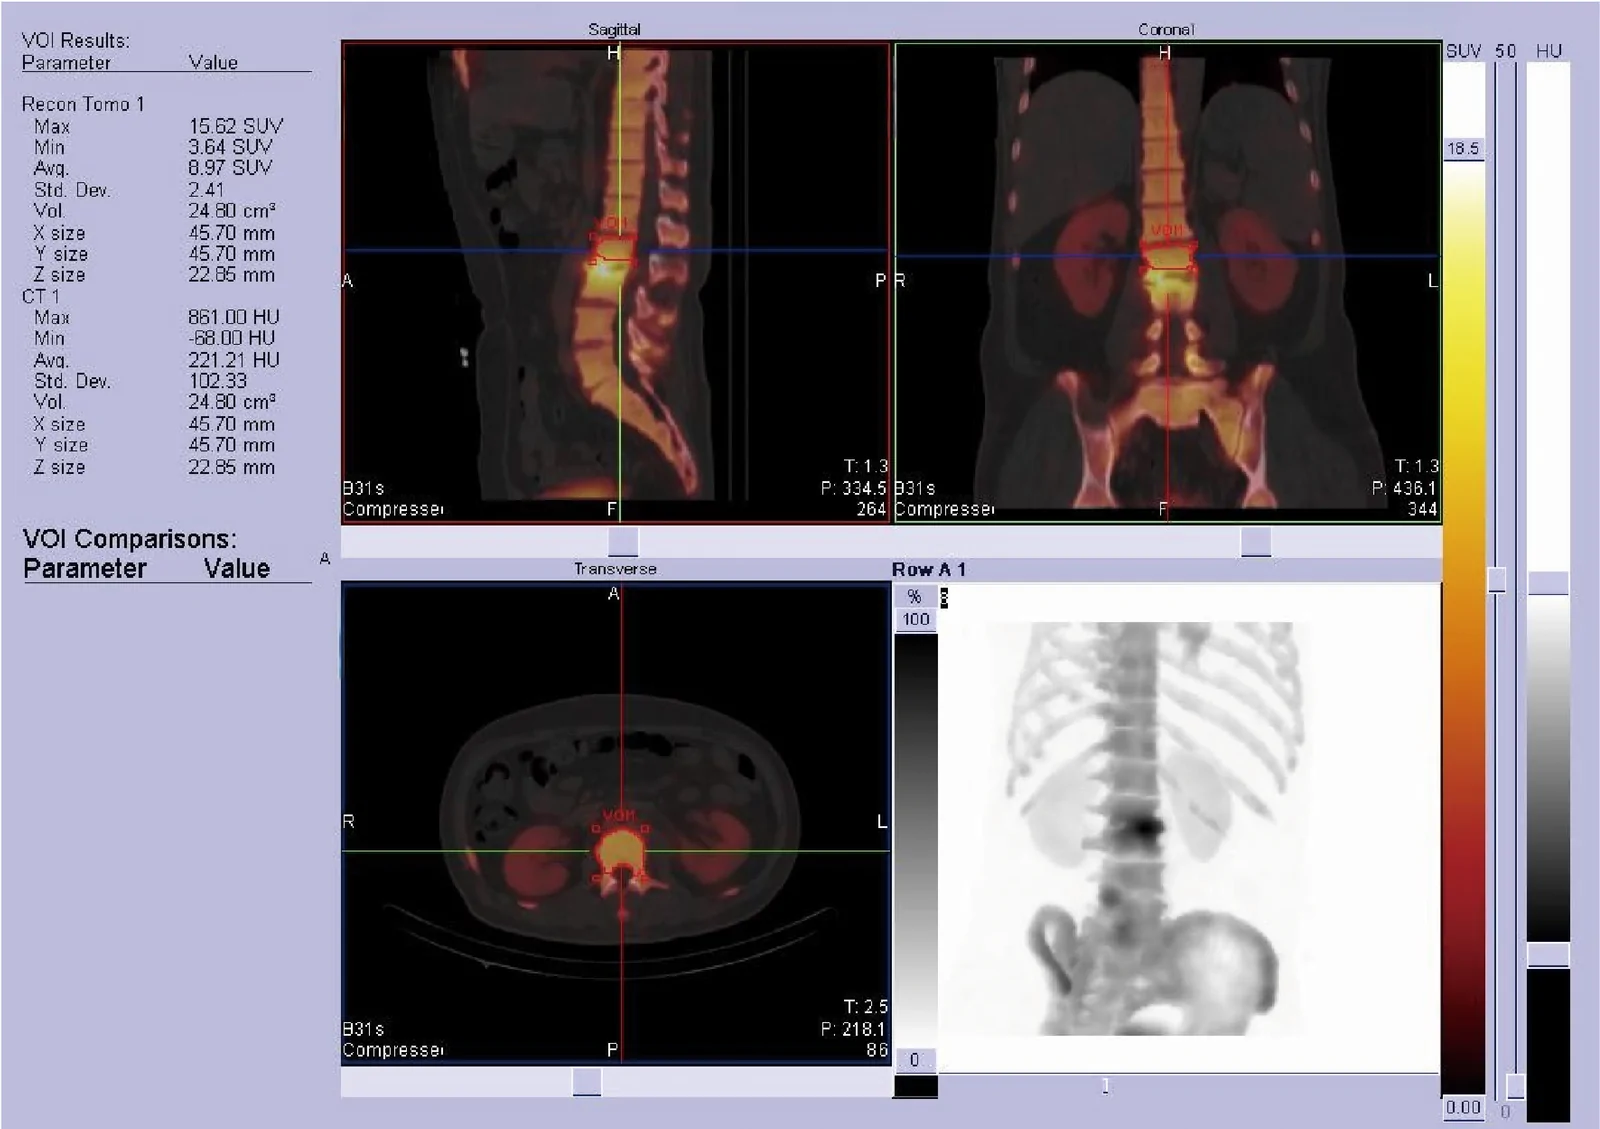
Quantitative SPECT/CT examination obtained in a patient with spinal tuberculosis involving the L2 and L3 vertebra. Fused SPECT/CT images in the sagittal, coronal, and axial planes, together with the corresponding planar bone scintigraphy image, demonstrate increased radiotracer uptake in the involved L2 and L3 vertebral lesion.
